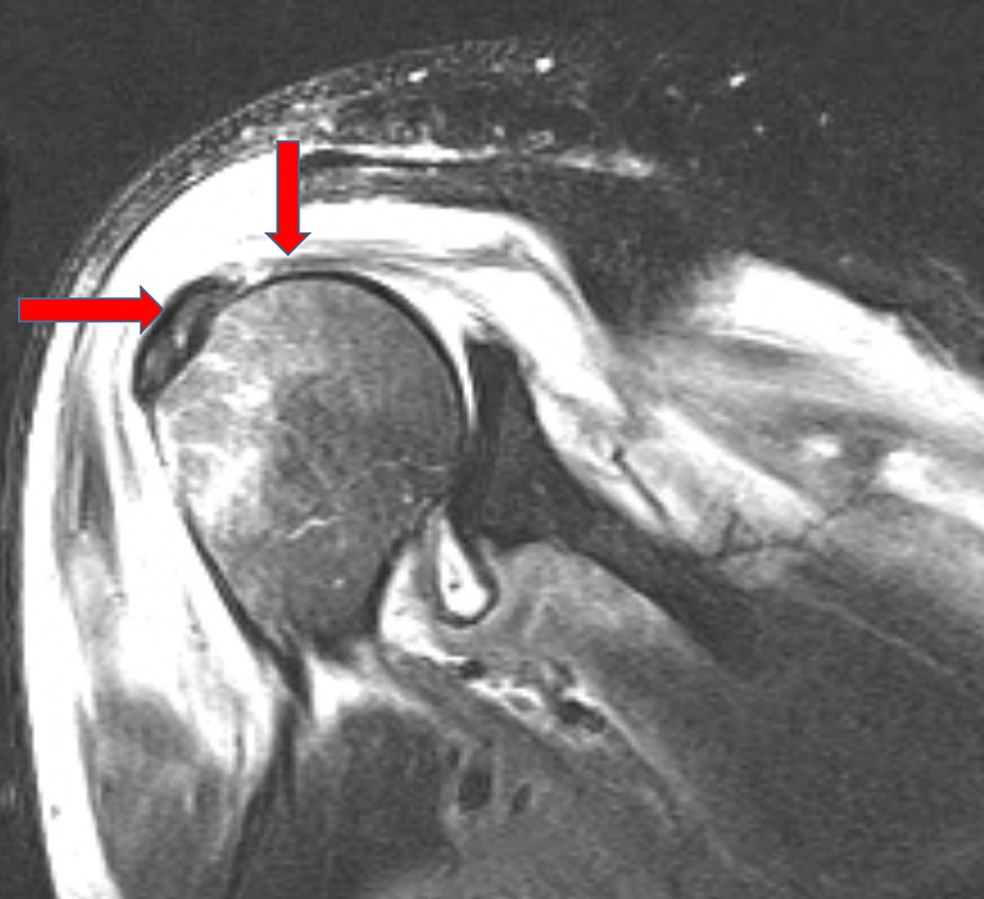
MRI of right shoulder: Moderate of fluid (as shown by the red arrows) within the subacromial-subdeltoid bursae as well as within the joint which represents septic arthritis. Diffuse edema especially within the infraspinatus muscle may represent infectious myositis or reactive changes.
